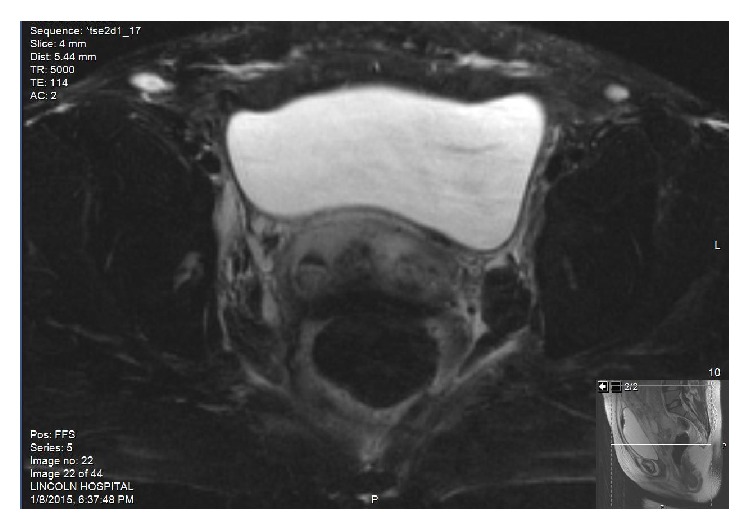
MRI of abdomen and pelvis with contrast: Series # 5, T2 axial FS: one cervix on the right and one cervix on the left, 2 separate cervices.
